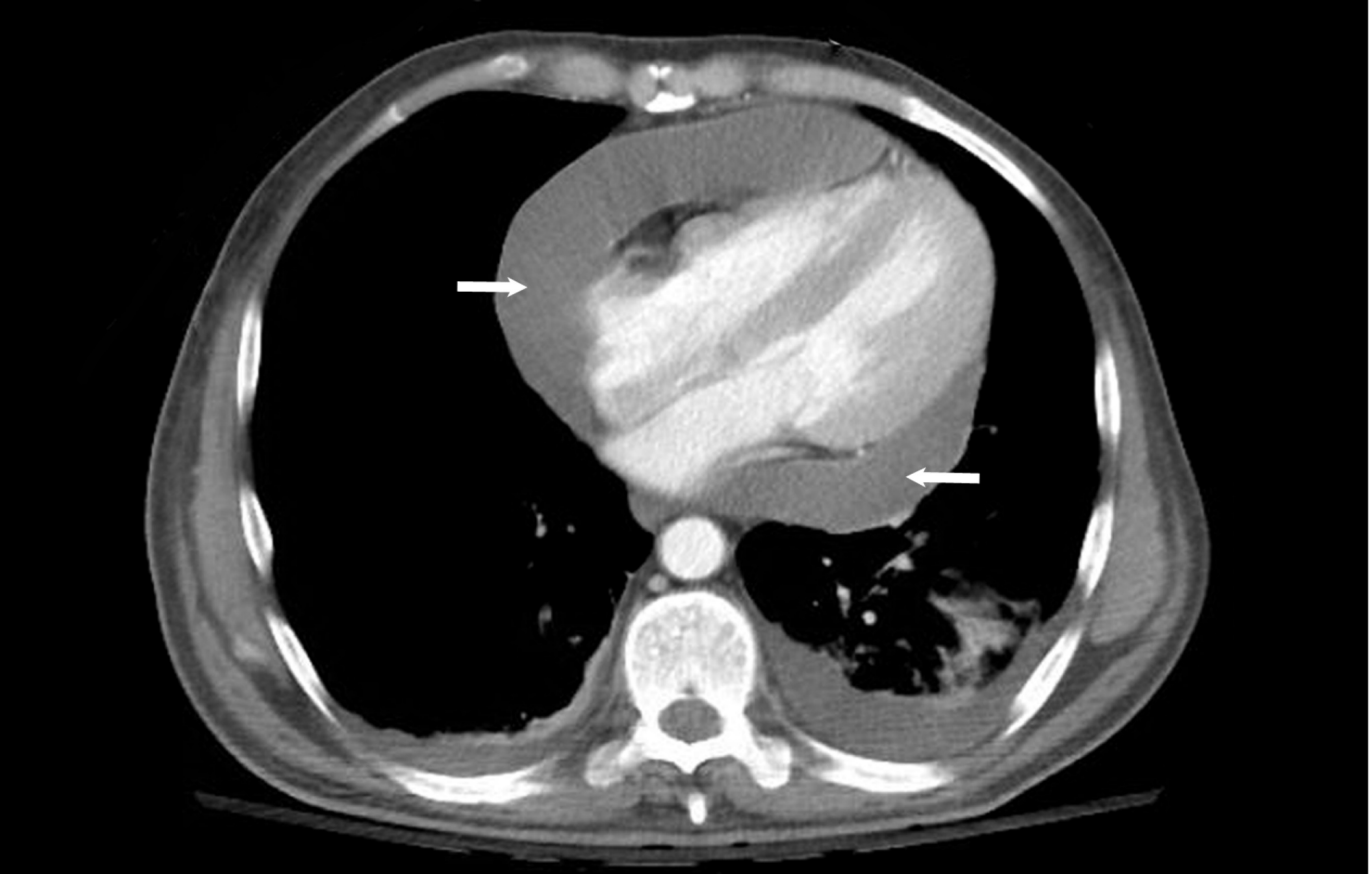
Computed tomography. Computed tomography portraying circumferential pericardial effusion (arrows).
